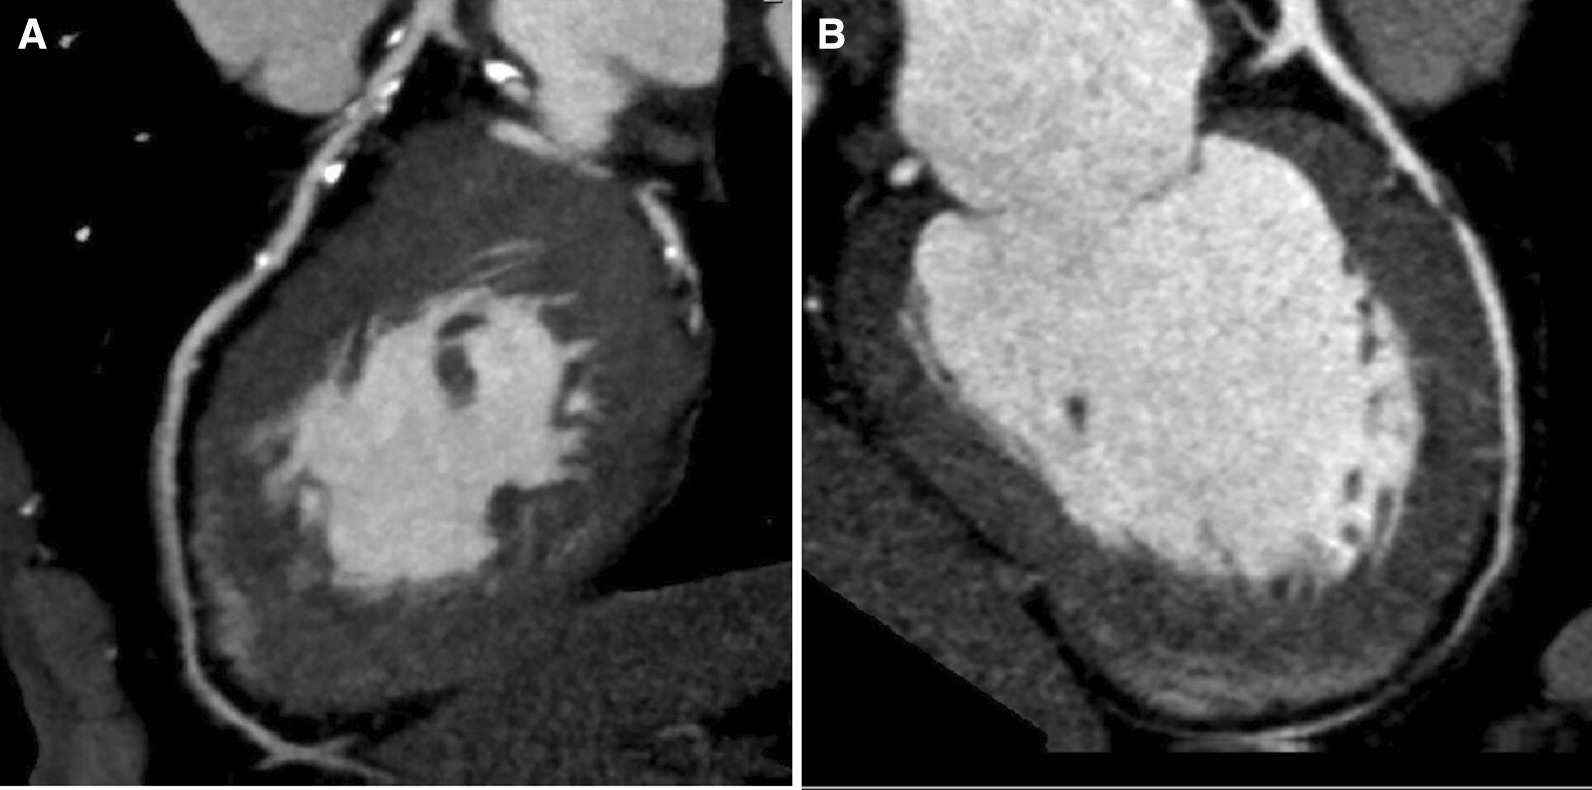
A patient with clacified plaque (A). Coronary CT angiography showed proximal LAD calcified lesion in a patient with total coronary calcium score 86 HU. A patient with soft (non-calcified) plaque (B). Coronary CT angiography showed proximal LAD non-cacified lesion in a patient with total coronary calcium score zero
